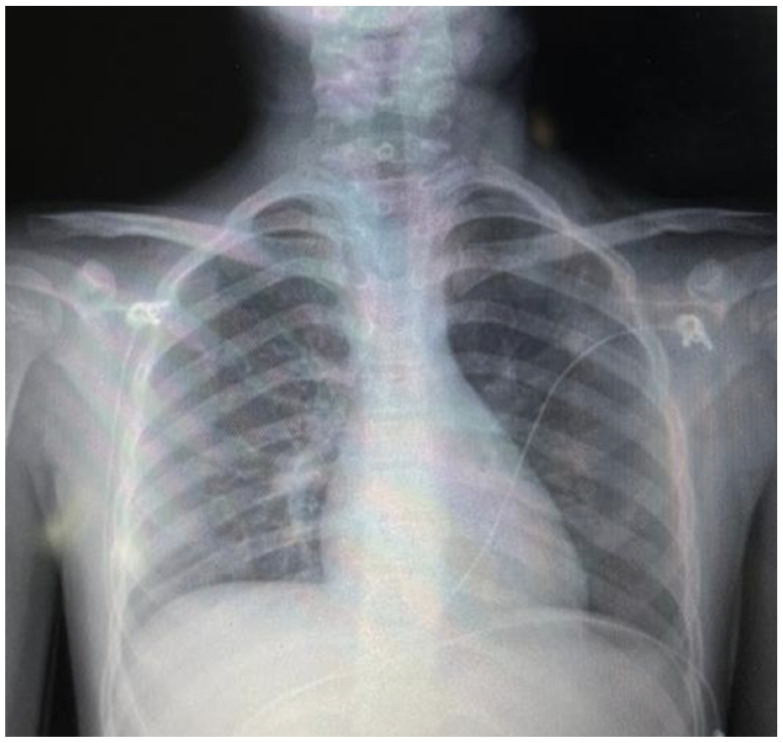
Follow-up posteroanterior chest radiograph (hospital day 3, pre-discharge) demonstrating near-complete resolution of subcutaneous emphysema and pneumomediastinum compared with the admission study (Figure 1). The cardiac and mediastinal contours are now clearly delineated without surrounding radiolucent halos.
